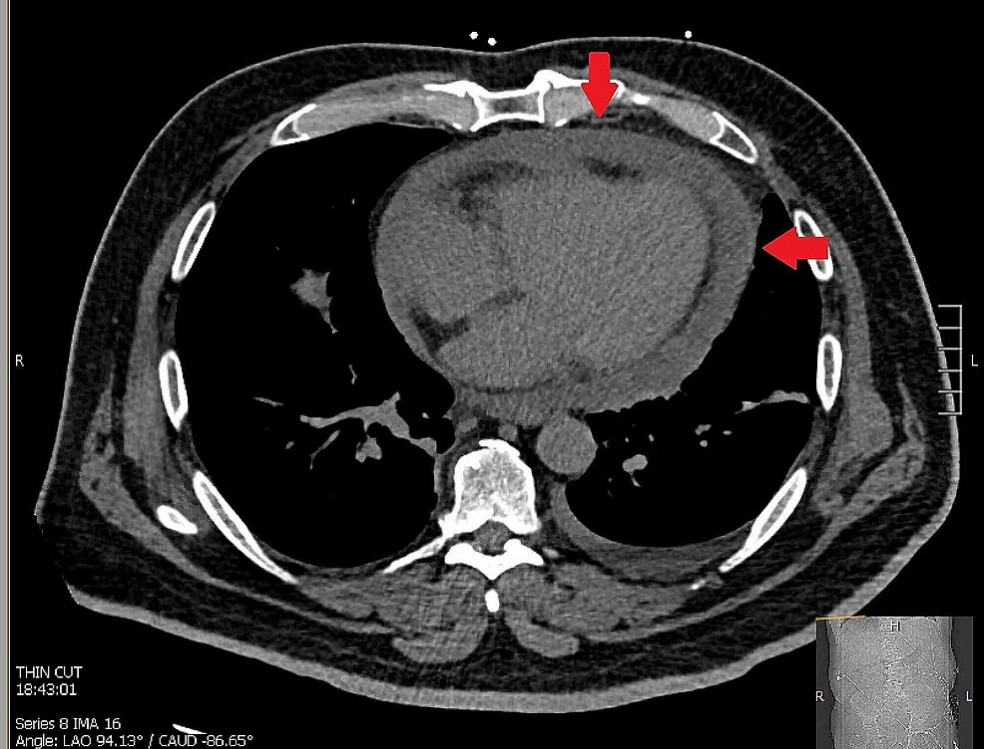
CT scan of the chest with arrows indicating a large, circumferential pericardial effusion. CT = Computed tomography
